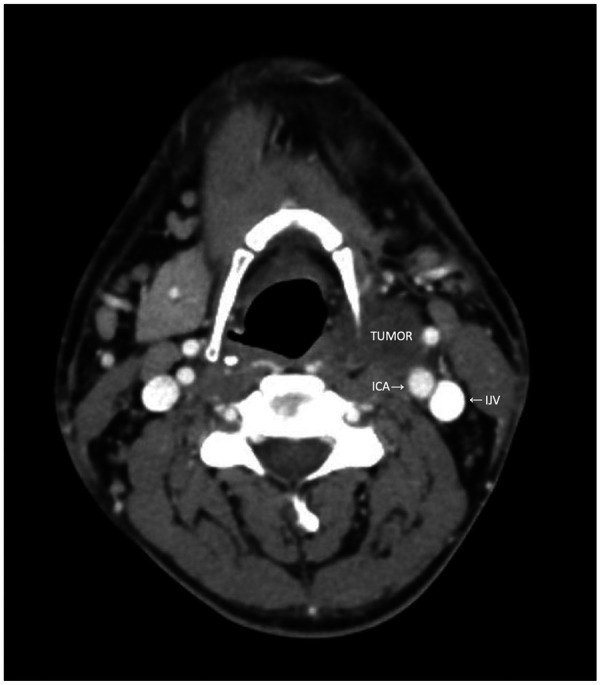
Imaging findings of a sympathetic chain schwannoma. Axial contrast-enhanced CT image of the neck shows a post-styloid parapharyngeal space mass displacing the internal carotid artery (ICA) and internal jugular vein (IJV) together laterally without clear separation between the two vessels. This pattern was interpreted as suggestive of sympathetic chain origin, which was confirmed intraoperatively.
